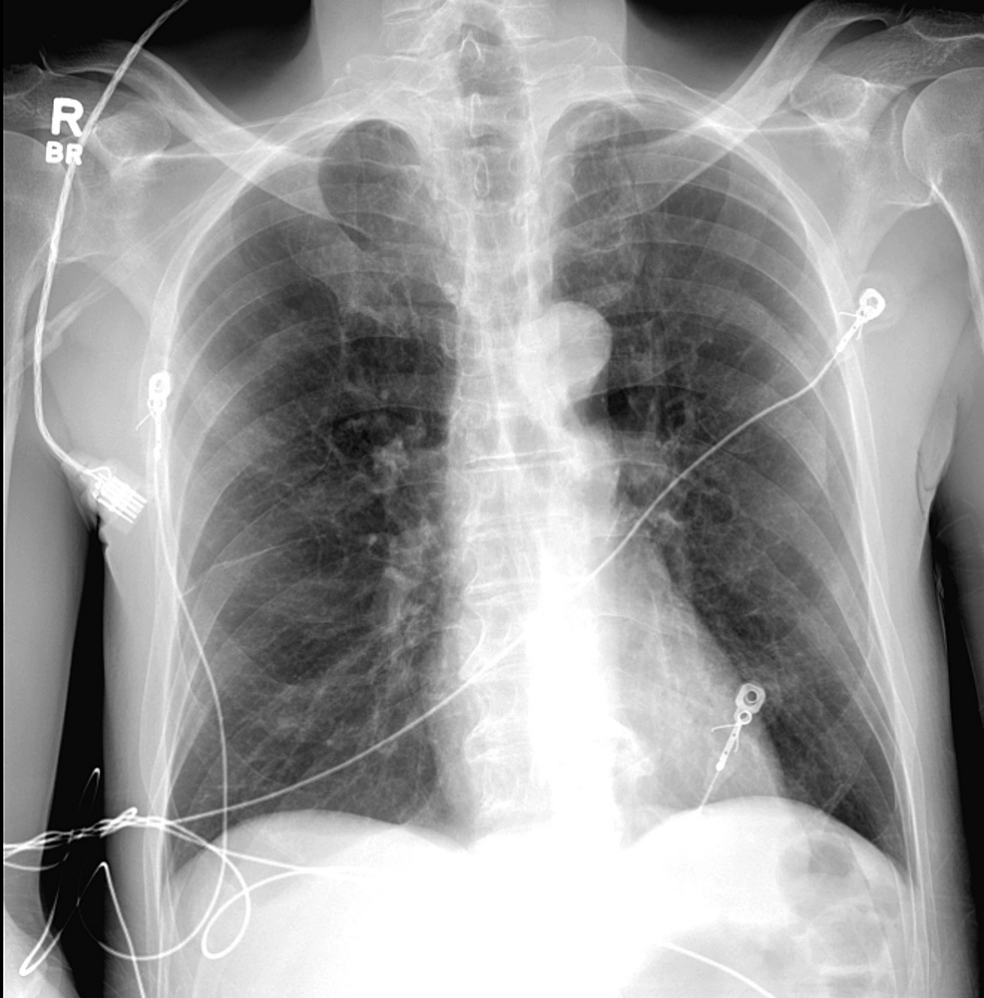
CXR post-biopsy without PTX CXR: chest radiograph; PTX: pneumothorax
